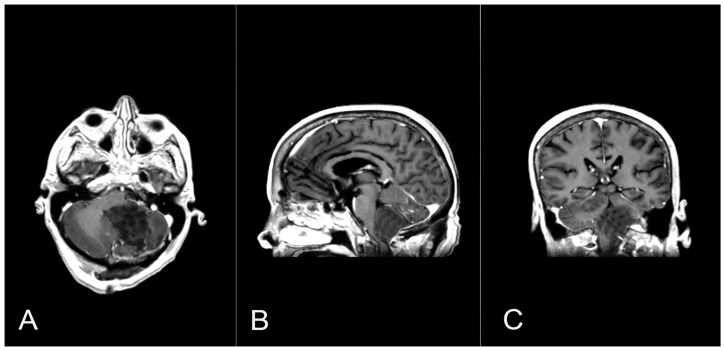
Axial (A), sagittal (B) and coronal (C) postoperative T1 contrast-enhanced brain MRI shows the left suboccipital craniectomy accomplished and the GTR exeresis of the lesion.
